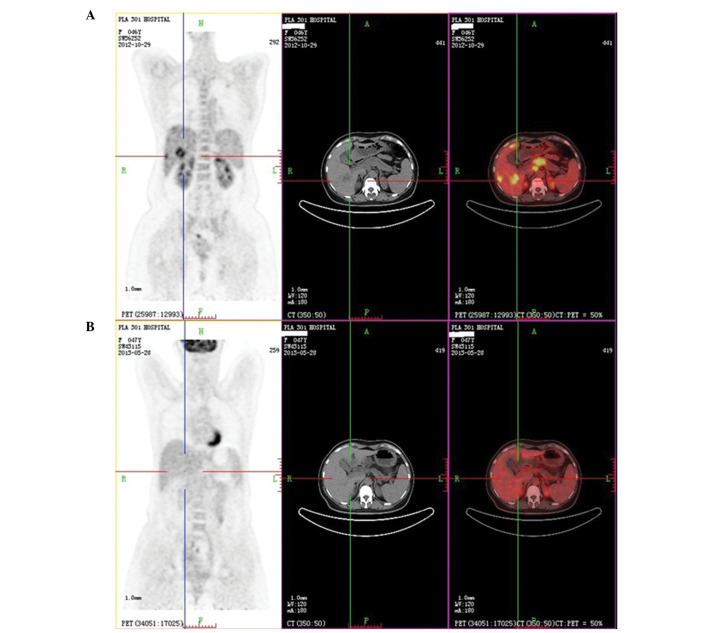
Positron emission tomography/computed tomography of the patient prior to the first cycle of chemotherapy plus targeted therapy on (A) Oct 29th, 2012 and (B) following the eighth cycle of chemotherapy plus targeted therapy combined with 14 infusions of expanded activated allogeneic lymphocyte therapy on May 28th, 2013. The metabolic values of the deposits in the pancreas, liver and abdominal lymph nodes decreased markedly and even disappeared. In addition, the deposits in the lungs decreased significantly, along with the size of all the lesions compared with the lesion sizes prior to treatment.
